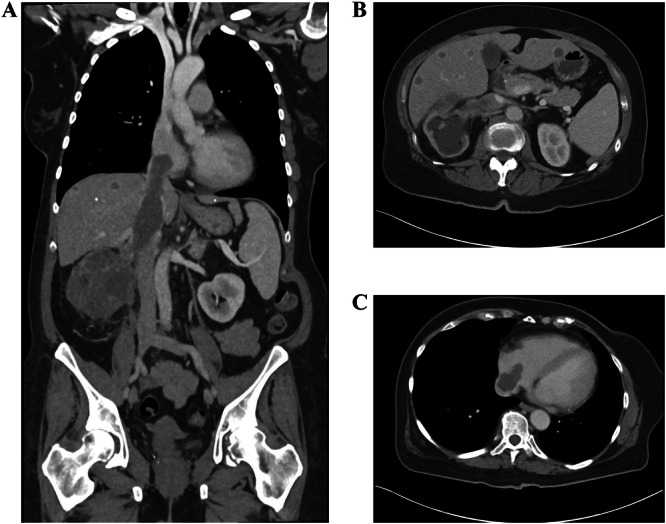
Computed tomography of a patient with renal cell carcinoma and Neves IV venous tumor thrombus. (A) Coronal view. (B) Axial view at level of renal vein. (C) Axial view at level of right atrium.
